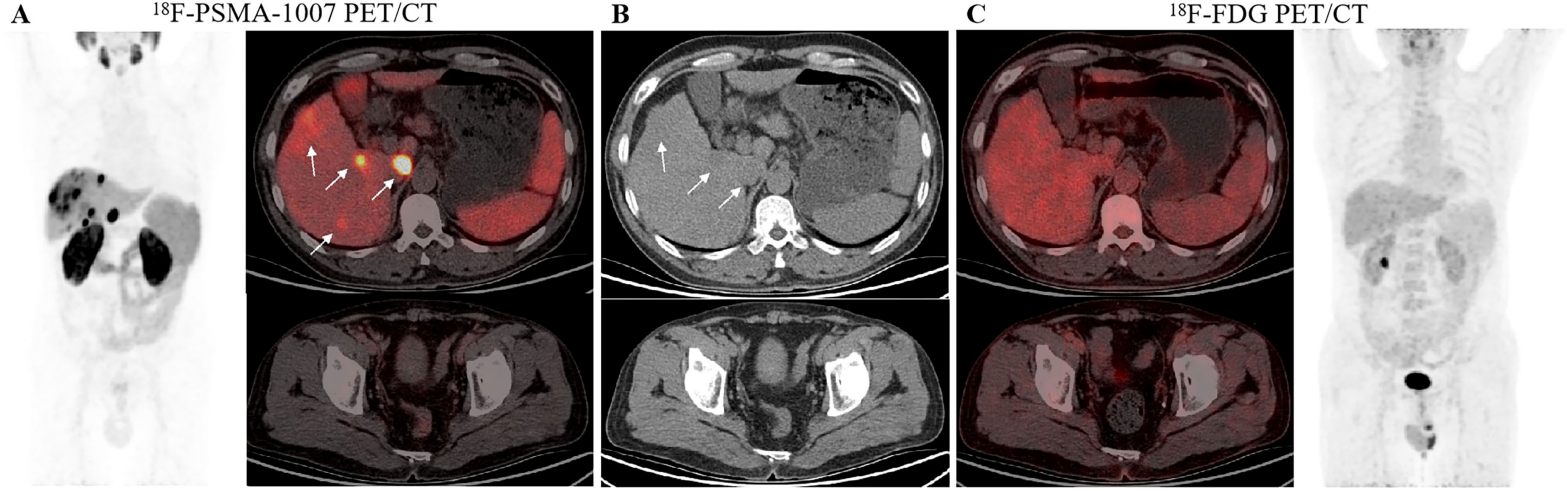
Comparison of ^18^F-PSMA-1007 and ^18^F-FDG PET/CT imaging in a 63-year-old man with HCC (arrow indicates lesion). (A) Maximum-intensity projections (MIP) of PET using ^18^F-PSMA-1007 and transversal fusion images of primary hepatocellular carcinoma and right medial gluteal muscle (T: 71.6, L: 7.6, T/L: 9.4; A: 1.1, T/A: 65.1; M: 0.5, T/M: 143.2). (B) Transverse CT images revealed low-density masses in the right lobe of the liver and right medial gluteal muscle (C) MIP of PET using ^18^F-FDG and the radiation uptake of HCC and right medial gluteal muscle (T:3.4, L:3.5, T/L: 1.0; A: 2.1, T/A: 1.6; M: 1.1, T/M: 3.1).
